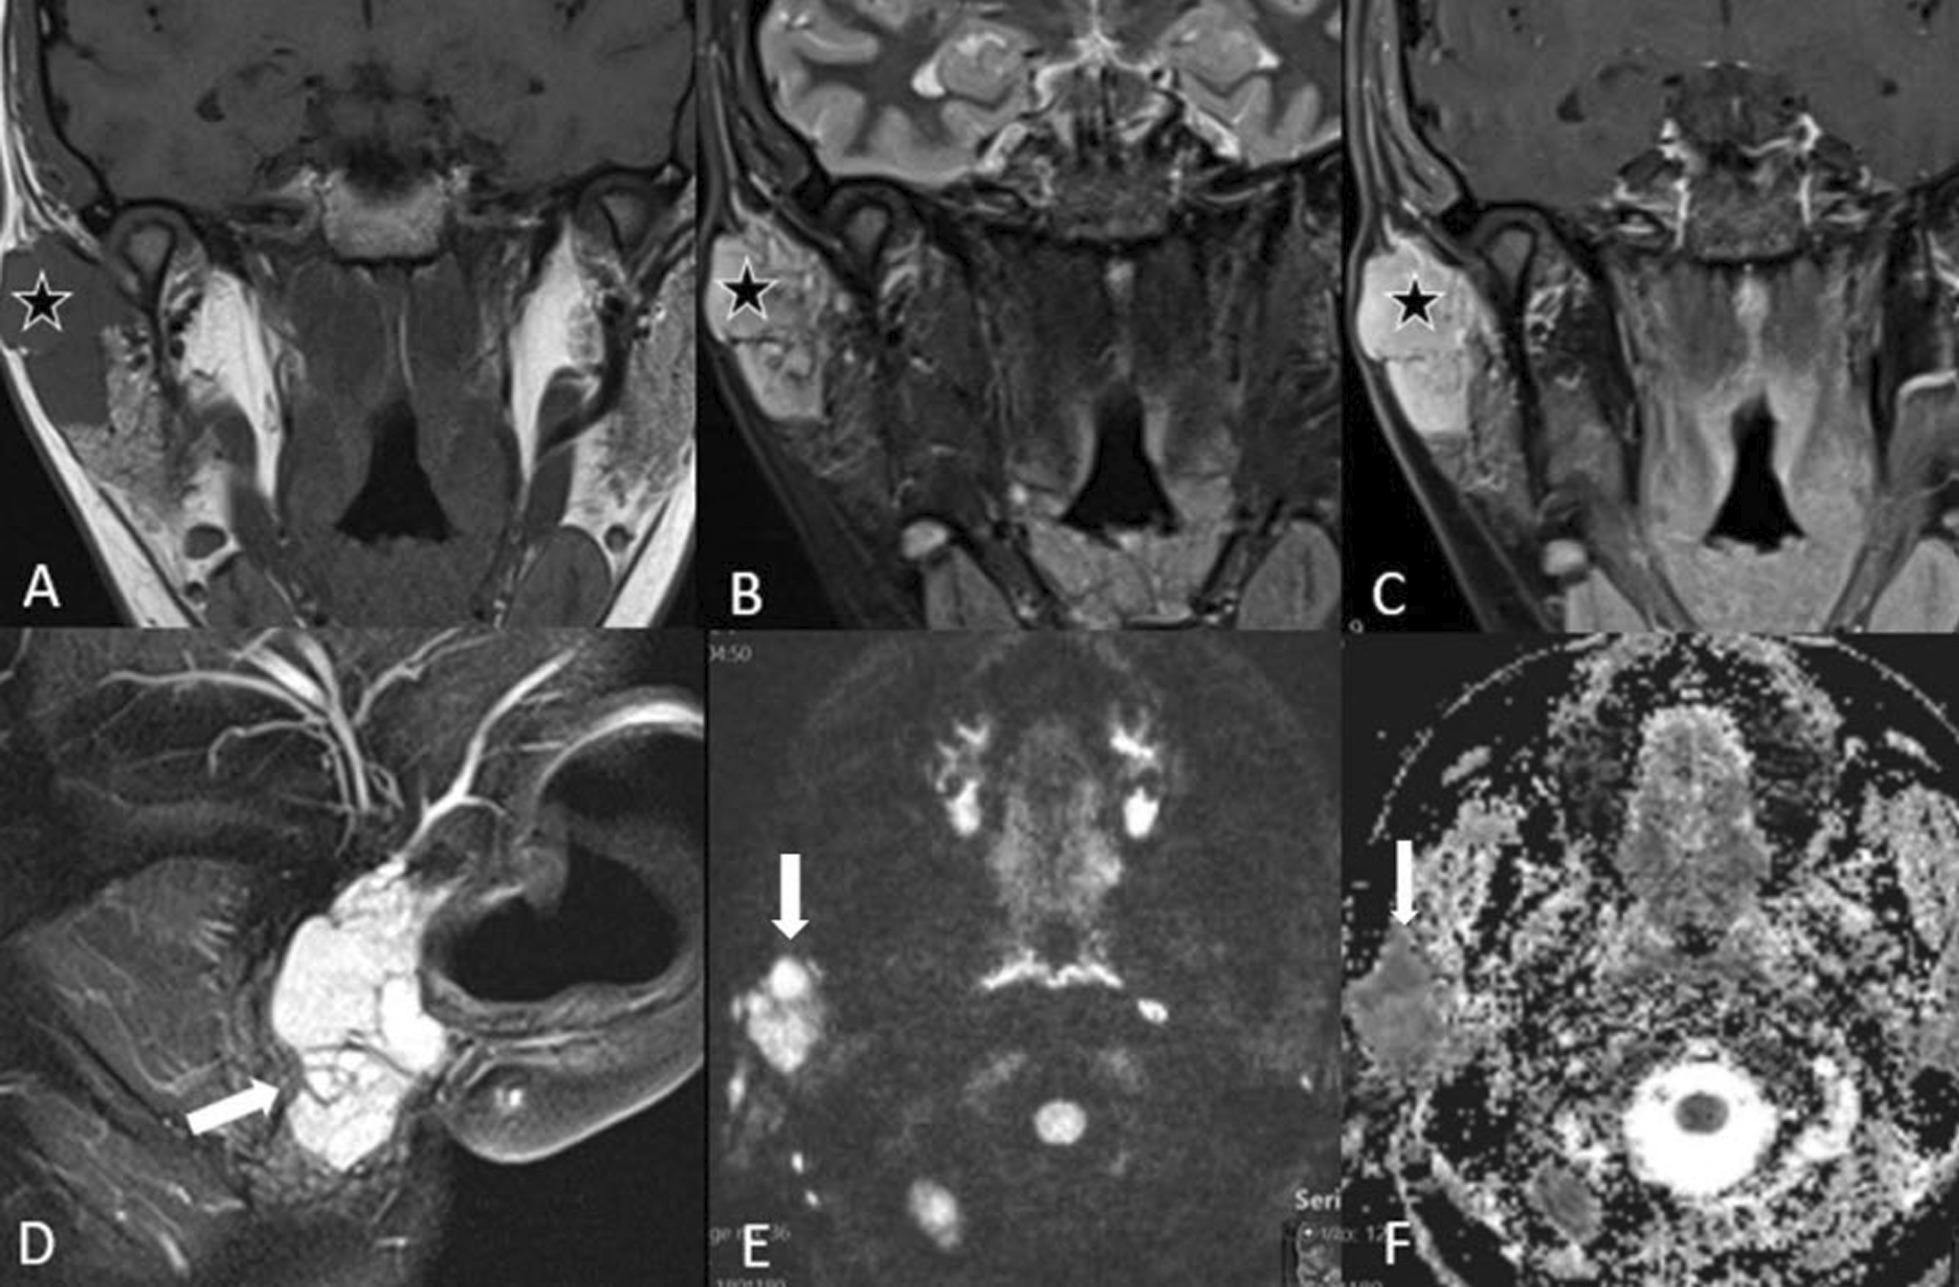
Adenoid cystic carcinoma. A 38-year-old female presented with a painful preauricular swelling on the right side. Coronal T1-w MRI (A) shows a homogeneously hypointense mass (star) in the superficial lobe of the right parotid gland, which is hyperintense on the coronal T2-STIR MRI (B) and shows strong and homogeneous enhancement on the post-contrast T1-w image with fat suppression (C). The lesion is lobulated (arrow) as seen in the sagittal T2 image through the parotid gland (D). The lesion shows high signal intensity (arrow) on the high b value image (E) with a corresponding low signal (arrow) on the ADC map (F) indicating diffusion restriction. The parotid gland on the left side does not show any increase in signal intensity in the high b value image (E)
